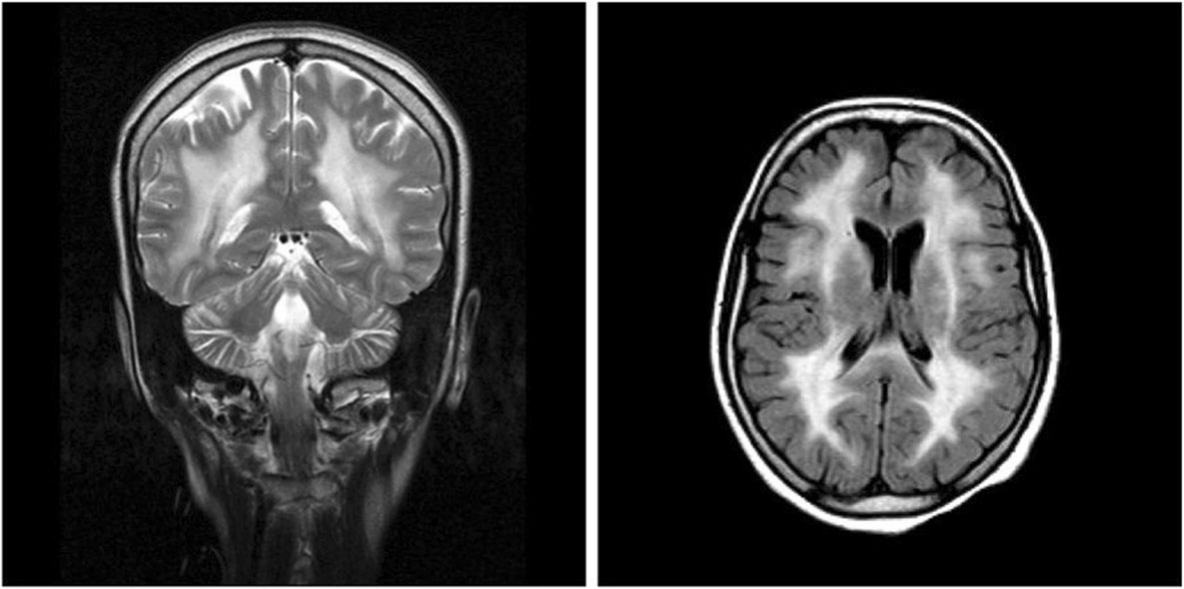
Brain MRI revealing diffuse white matter signal changes
